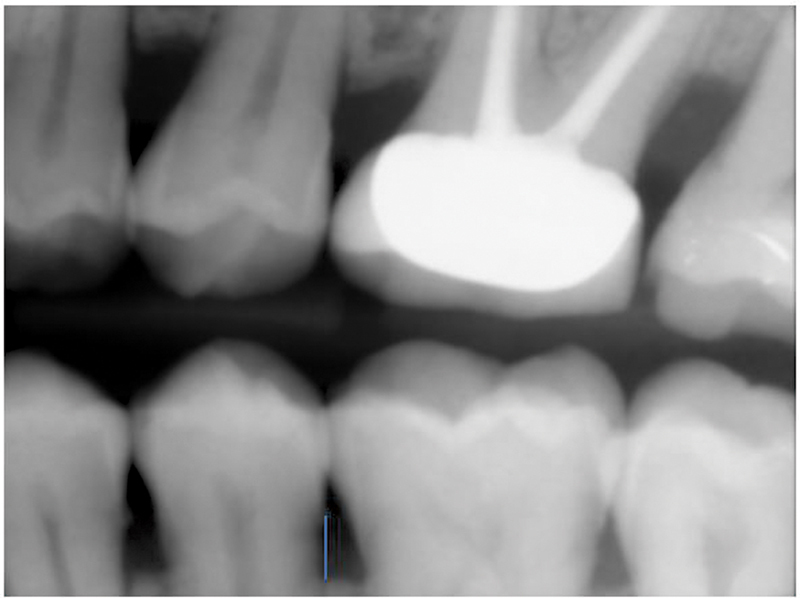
A bitewing radiographs of the patient for alveolar bone loss measurements. A straight line was sketched on the mesial and distal side of the tooth parallel to the long axis of each tooth from the most apical part of cementoenamel junction to the alveolar crest to measure the mean alveolar bone loss. A blue line is drawn at the distal aspect of the second premolar.
